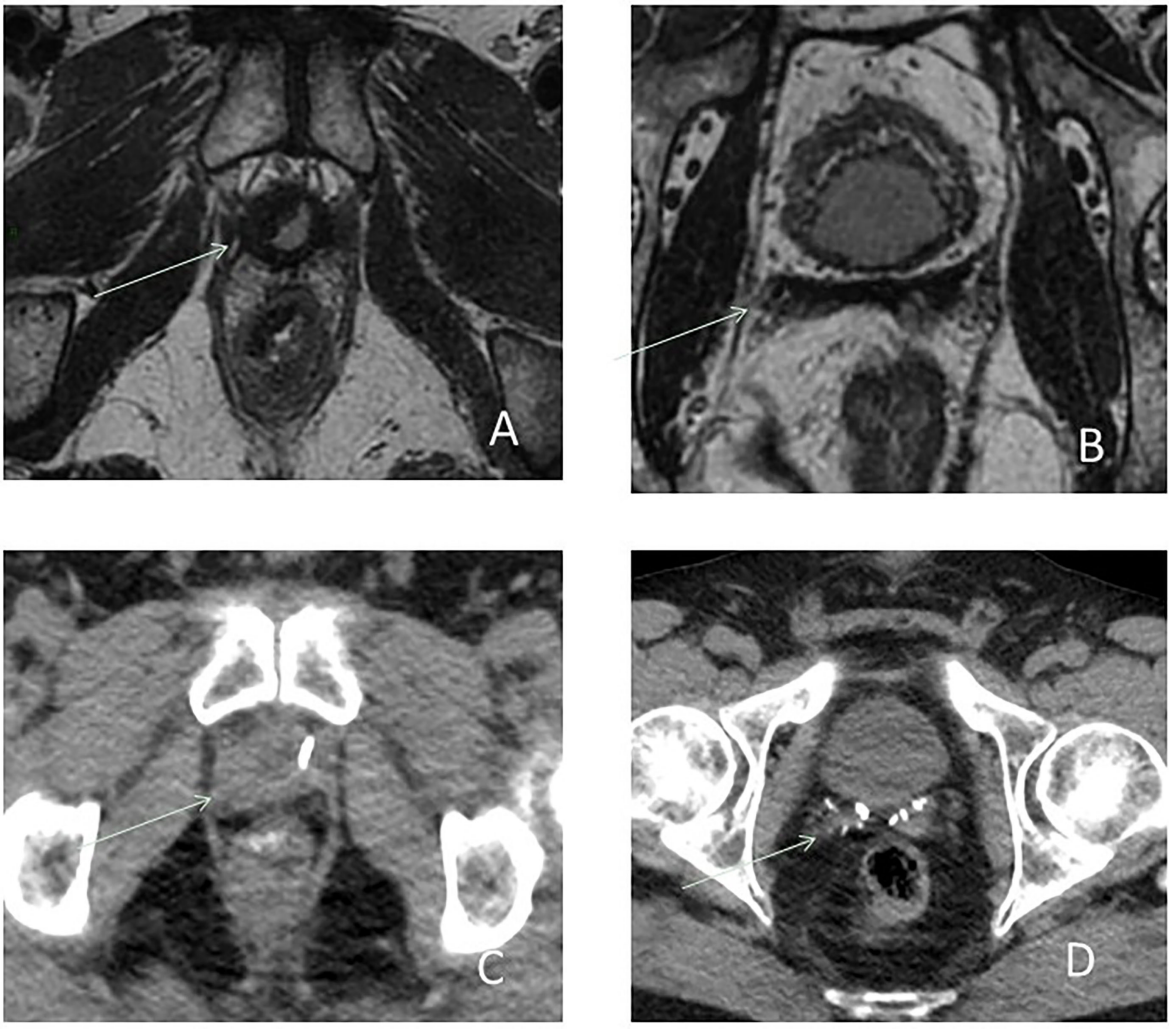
Biological recurrence in a 58-year-old male. Axial T2-weighted images (A, B) and CT (C, D) showing the benefit of MRI to enable a better delineation of anatomical analysis of the VUA (A, C), cut end of the vas deferens and SV bed (B, D), and bladder neck, all common sites of recurrences.
